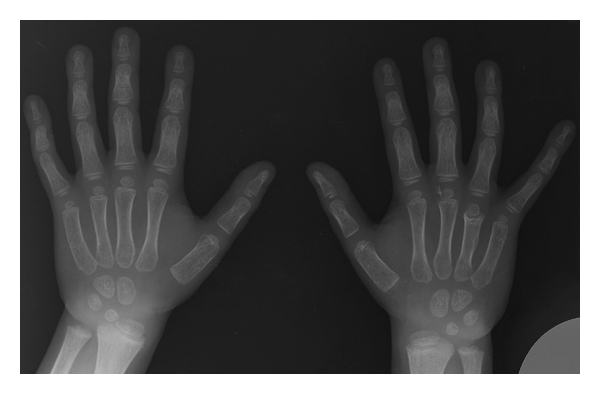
3-year posttreatment radiographs showing discrete shortening of the right hand fourth metacarpal.
